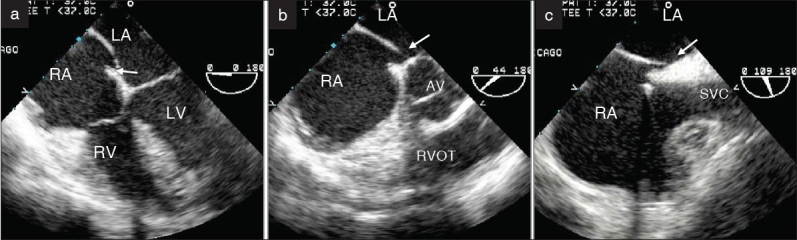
Transesophageal evaluation of PFO. (a) Four-chamber view to evaluate the atrioventricular valves and superior rims. (b) Aortic short-axis view to evaluate the aortic and the posterior rim and (c) Bi-caval view to evaluate the superior vena cava and the inferior vena cava rims. Note that the septum primum (the thin septum) bulges into the left atrium in the four-chamber view
